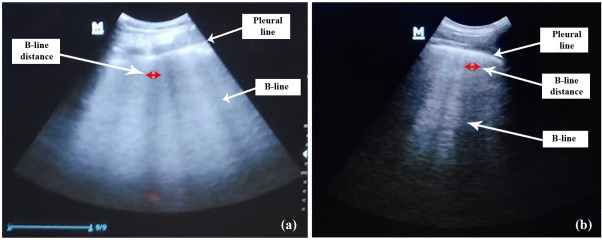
Lung ultrasound images demonstrating typical B-line patterns in RA-associated interstitial lung disease: (a) Multiple coalescent vertical hyperechoic artifacts (B-lines) with narrow inter–B-line spacing (B3), consistent with a NSIP pattern. (b) Multiple vertical hyperechoic artifacts (B-lines) with wider inter–B-line (B7), consistent with a UIP pattern. NSIP: nonspecific interstitial pneumonia; UIP: usual interstitial pneumonia.
